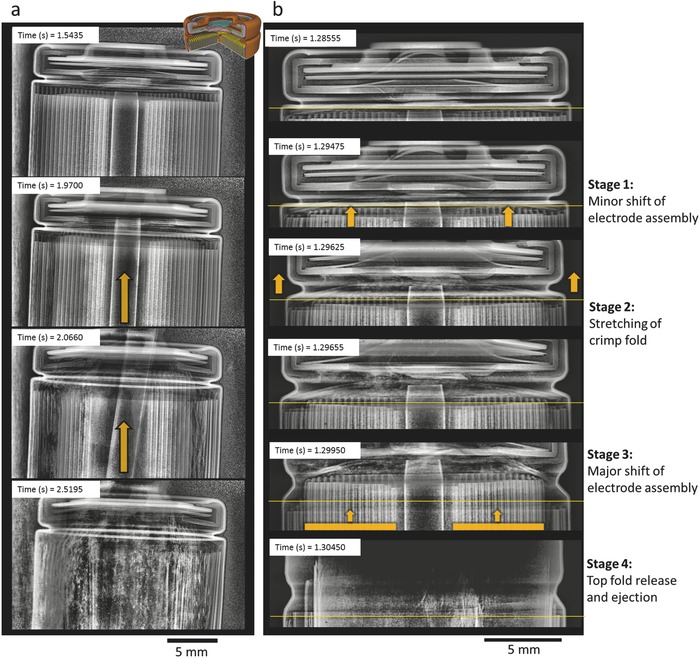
Time‐stamped radiographs taken at a) 2000 fps showing the mandrel piercing the crimp‐components during thermal runaway where the yellow arrows highlight the direction of shift of the mandrel, and b) taken at 20 272 fps showing the stages of the 18650 Panasonic cell bursting. Radiography movies of (a) and (b) are provided as Movies S12 and S13, respectively, in the Supporting Information.
